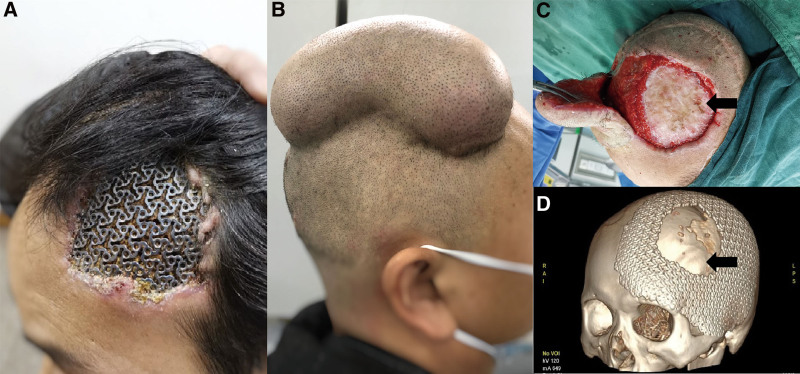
(A) Physical examination at admission revealed a frontotemporal scalp defect and titanium mesh exposure (12 cm × 10 cm). (B) A kidney-shaped tissue expander was implanted under the vertex scalp at the first stage of reconstruction surgery. (C) Removing the exposed part of the implant revealed a continuous epithelial layer (full-thickness skin with hairs) underneath (black arrow). (D) Postoperative 3D-CT demonstrated the removed part of the implant (black arrow).
